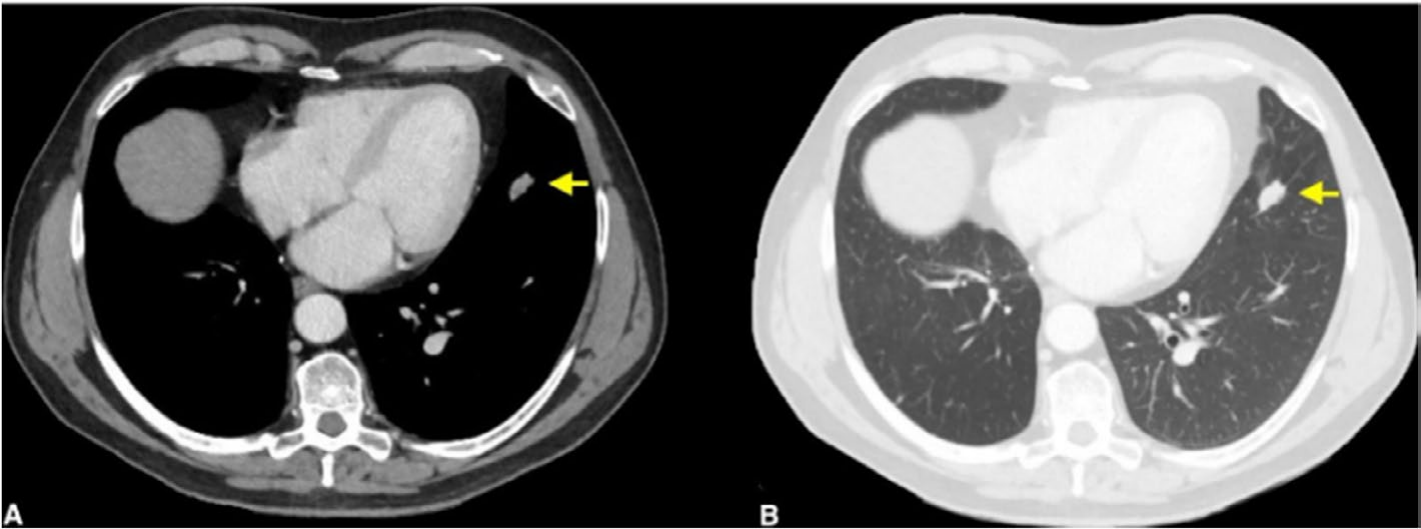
Chest CT scan showing a dense nodule of 20 × 14 mm at the inferior lingular segment. (A) Mediastinal window; (B) parenchymal window.
